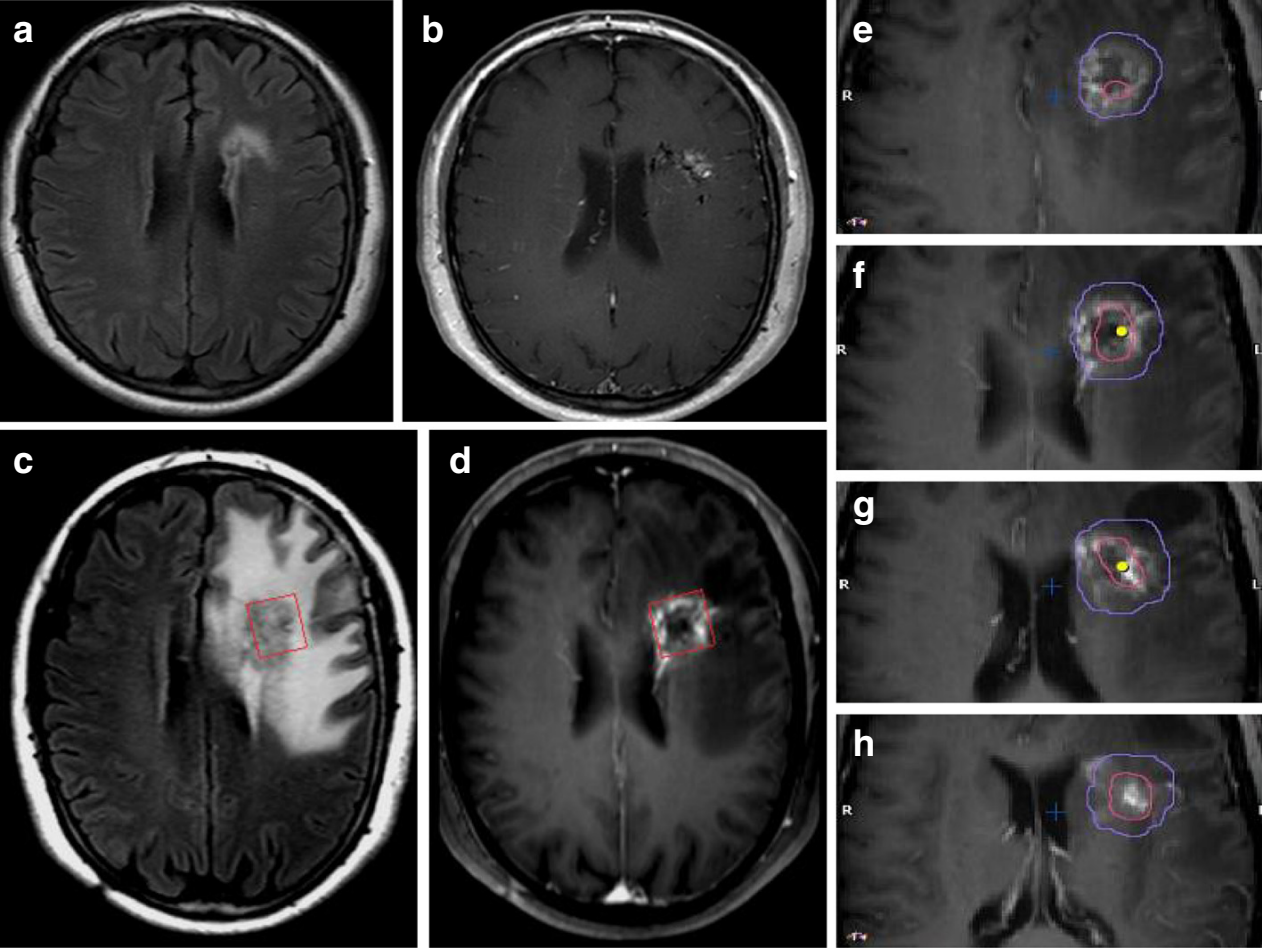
Same patient as Figure 1. Pre- and post-treatment MRI (FLAIR: a,c; contrast-enhanced MRI: b,d), e-h: fused post-treatment MRI). Red frame in c,d indicates selected volume for SV-MRS. Red line in e-h corresponds to PTV, purple line in e-h represents 10 Gy isodose line.
